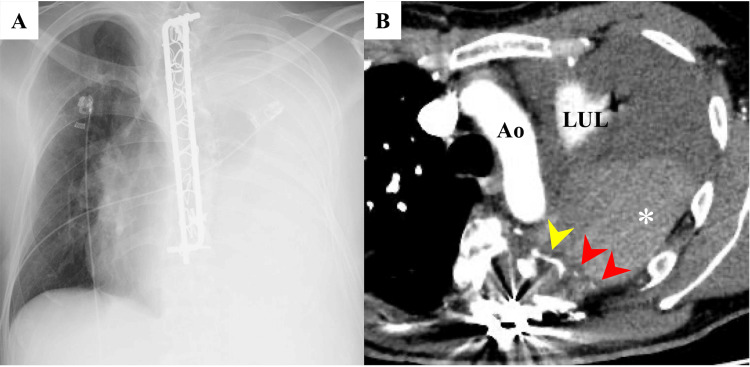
Chest X-ray (A) and chest computed tomography (B) A: Chest X-ray showing an opaque left hemithorax caused by massive pleural effusion (hemothorax) on admission. Linear metallic opacities along the thoracic spine correspond to prior scoliosis instrumentation. B: Contrast-enhanced chest computed tomography revealing a clot in the left thoracic cavity (asterisk), a hematoma along the left margin of the vertebral body (red arrowhead), and a tortuous intercostal artery (yellow arrowhead). LUL: Left upper lobe, Ao: Aortic arch
